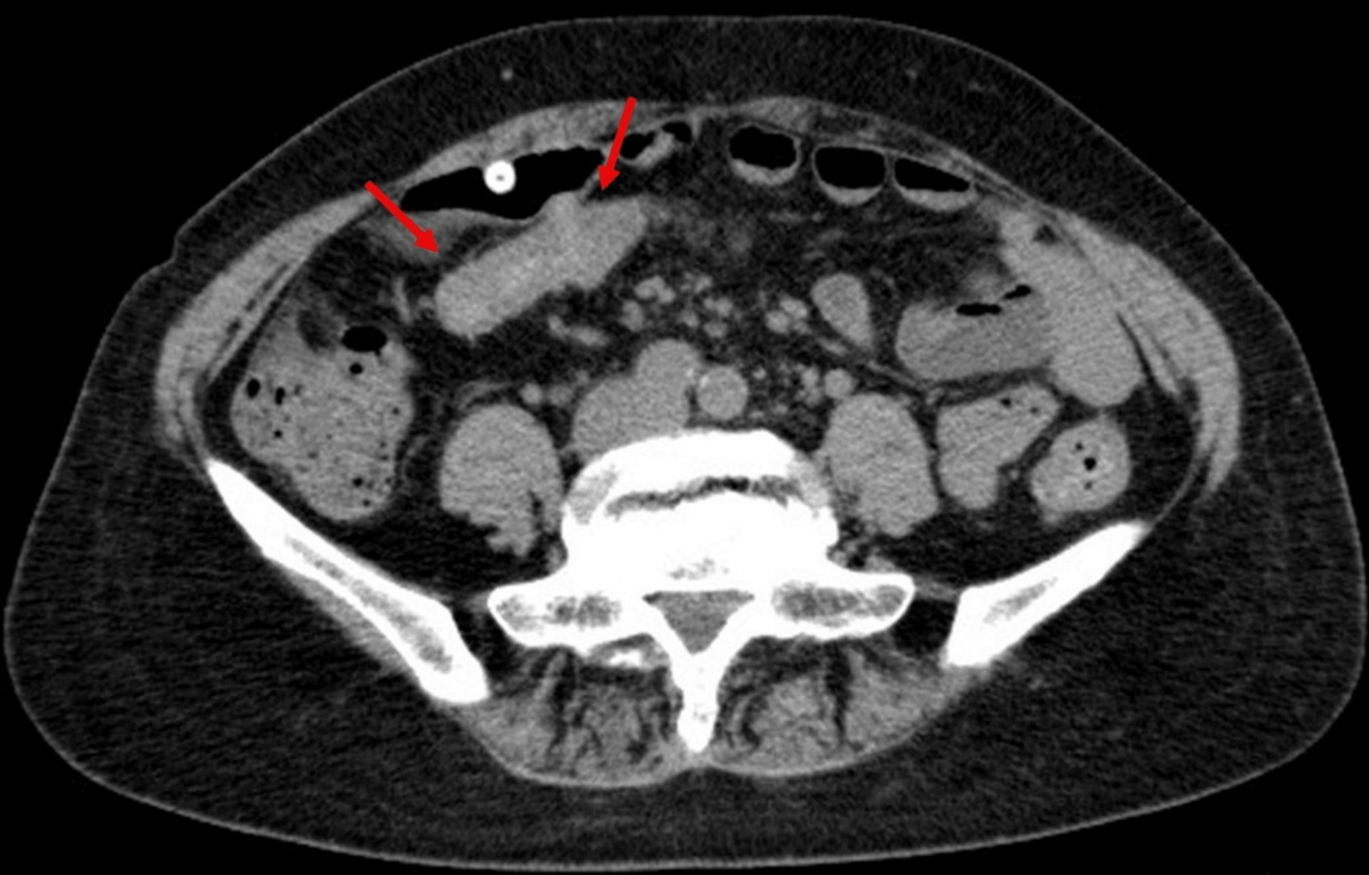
Abdominal CT findings. Edematous thickening is observed in the transverse colon (arrows). CT: computed tomography.
